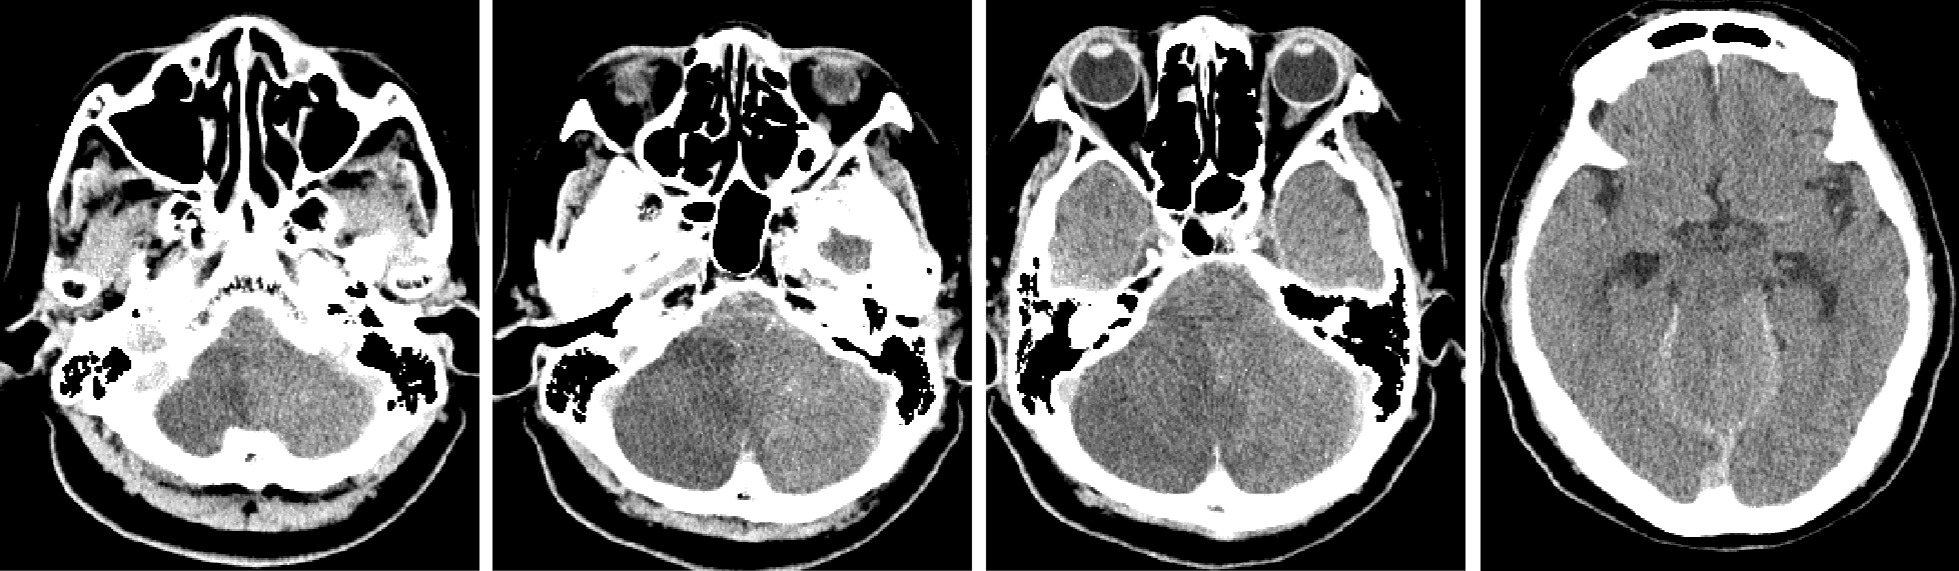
Postoperative brain CT demonstrated progressive infarction and transtentorial upward herniation
